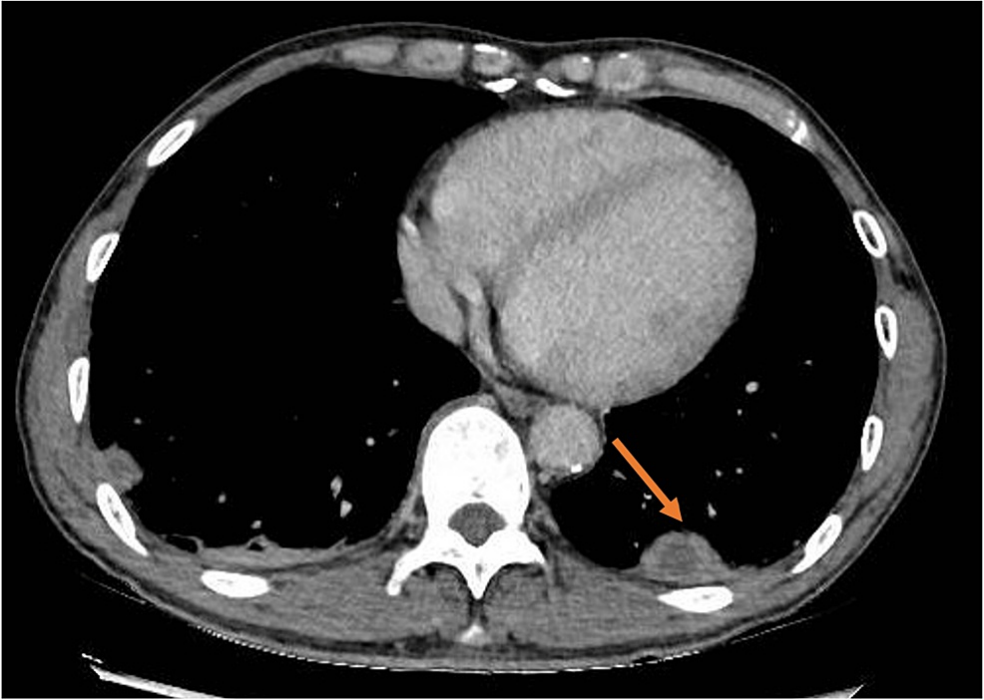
Computed tomography of the thorax showing a subpleural lesion with central necrosis in the left lower lobe.
